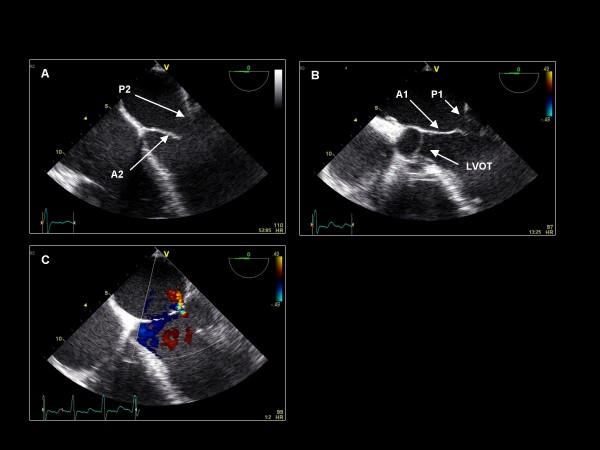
Imaging plane 0°, (A) The probe is advanced and retroflexed to remove the left ventricular outflow tract (LVOT) from view and demonstrate the A2 and P2 scallops of the mitral valve. (B) The probe is withdrawn and anteflexed to demonstrate the LVOT and the A1 and P1 scallops of the mitral valve. (C) Color Doppler applied to the mitral valve demonstrating very mild mitral regurgitation.
